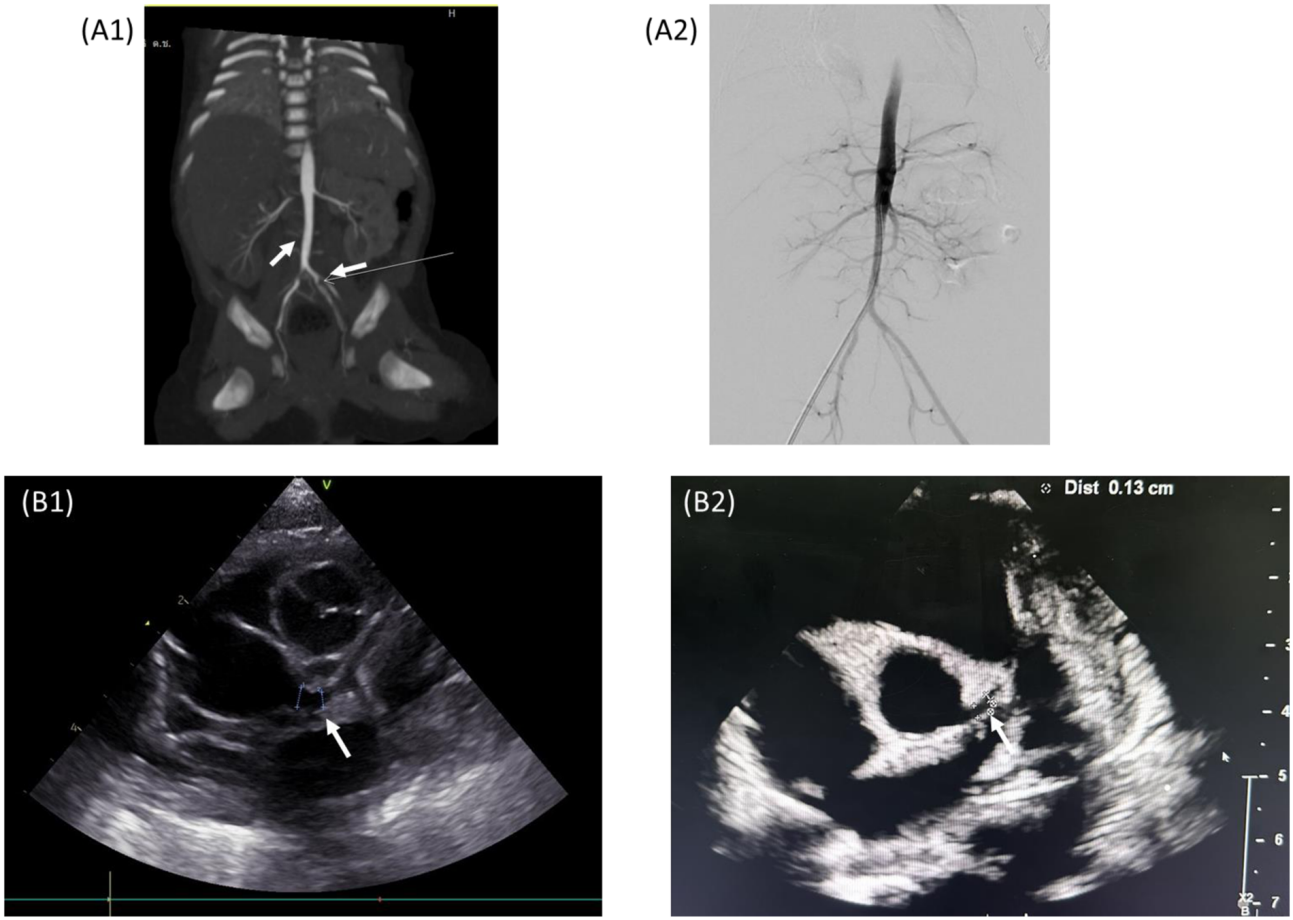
(A1) CTA abdominal aorta showing right renal artery stenosis and arterial thrombus in the juxta-renal abdominal aorta and left IIA. (A2) Angiography showing thrombus resolution at 3-week follow-up. (B1) Echocardiography at DOL 8 showing a new left coronary artery aneurysm. (B2) Echocardiography at age of 7 months showing regression of the coronary artery aneurysm to normal.
